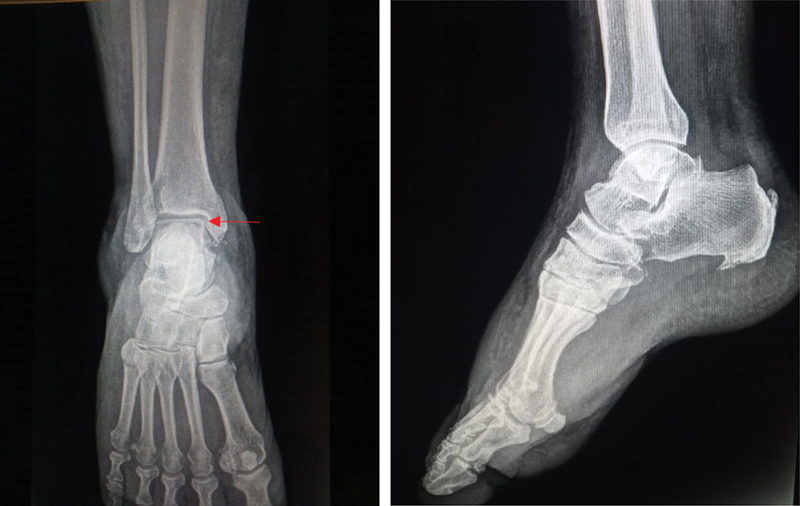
Radiographs revealed the Hawkins sign six weeks postoperatively (red arrow). No sclerosis or bone collapse of the talar dome was observed.
